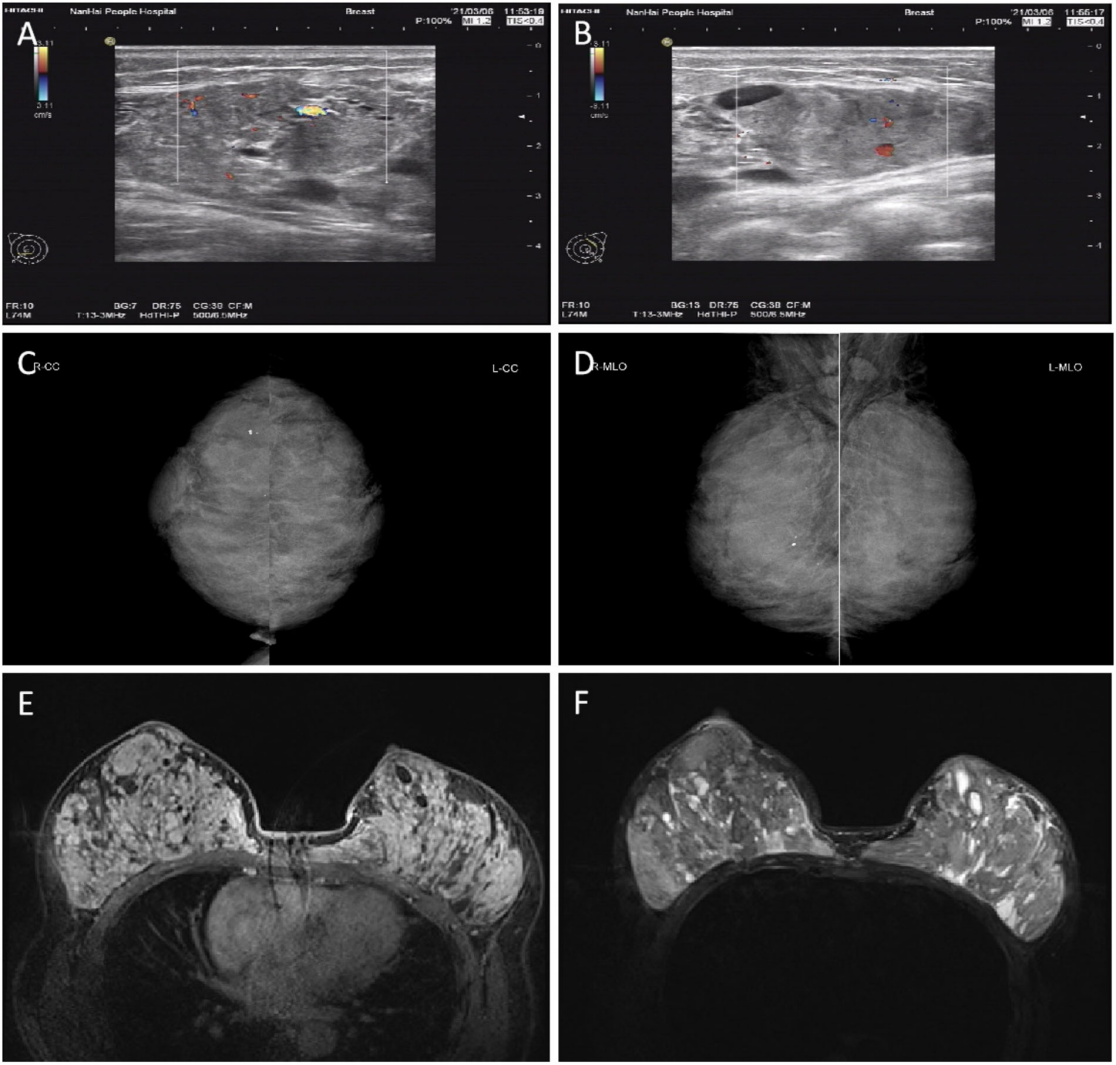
Preoperative color ultrasonography (A, B), mammography (C, D), and MRI (E, F) of bilateral breasts. Color ultrasonography (A, B) of the breast indicated a solid mass with a clear boundary, a low blood flow signal (A), and cystic changes. Mammography (C, D) indicated dense and lamellar bodies of both mammary glands, with no obvious nodular sensation, bilateral axillary lymph node shadows, and a few coarse calcifications in the right mammary gland. MRI (E, F) indicated dense glands in both breasts, with multiple mixed long T1 (E) and long T2 (F2) abnormal signal shadows in each quadrant. MRI, Magnetic resonance imaging.
